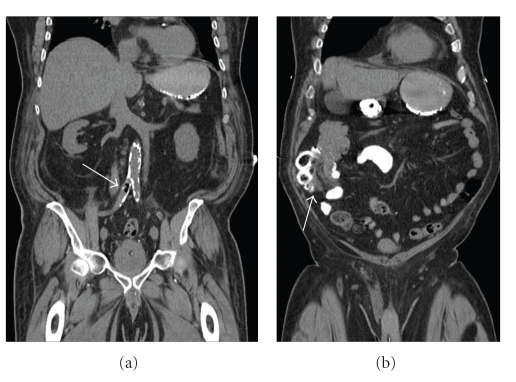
CT abdomen/pelvis (coronal): (a) multiple bubbles of gas within the lumen of the infrarenal aorta and right common iliac artery, consistent with bacterial aortitis and (b) ileo-colic intussusception caused by a cecal tubulovillous adenoma.
